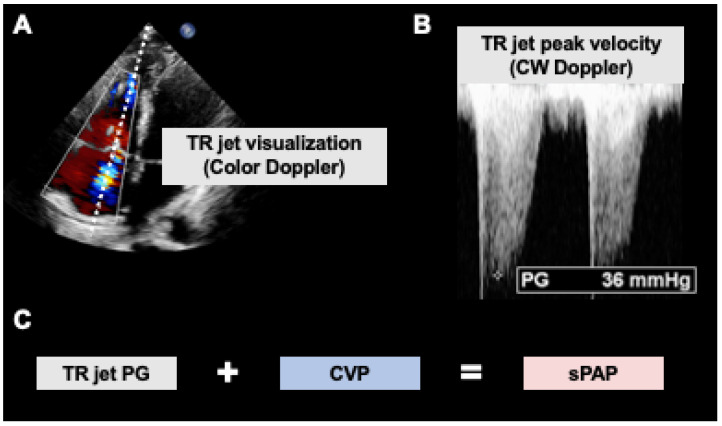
Noninvasive estimation of systolic pulmonary artery pressures using echocardiography. (A) Tricuspid regurgitation (TR) is visualized using the color Doppler mode in the four-chamber transthoracic view. (B) PW doppler recording of the TR jet yields its peak velocity during systole, which can be converted into a peak pressure (PG) by the modified Bernoulli equation (automatically). (C) The measured PG defines the difference in pressure between the right atrium and the right ventricle. Hence, central venous pressure (CVP) must be added to PG to derive the systolic pulmonary artery pressure (sPAP). The CVP can be read out invasively using a central line or estimated according to the collapsibility of the inferior vena cava.
